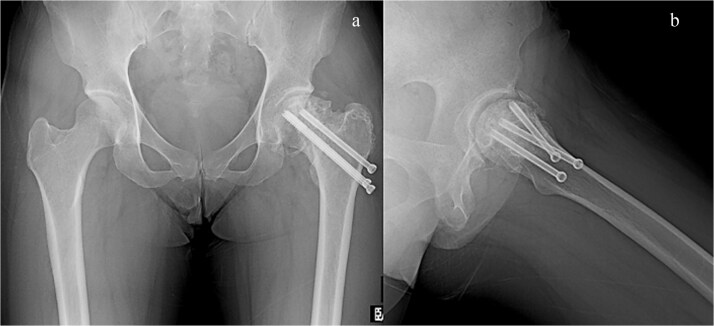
Plain radiograph at 10 years after surgery showed shortened union at the fracture site and mild osteoarthritic changes.
